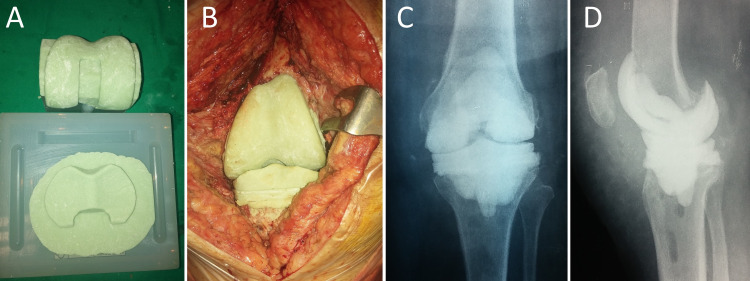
PJI of the left knee of a 75-year-old woman. A. Intraoperative preparation of the articulated ALCS on prefabricated molds. B. Implantation of the articulated ALCS after removal of the infected prostheses and debridement of the left knee. Anteroposterior (C) and lateral (D) radiograph of the left knee after the first-stage revision operation. PJI: prosthetic joint infection, ALCS: antibiotic-loaded cement spacer.
